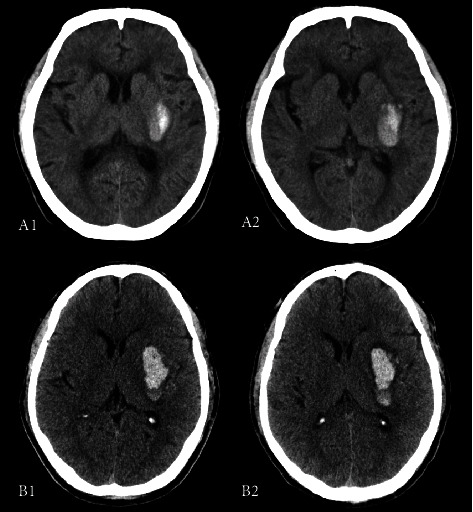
Basal ganglia hemorrhage. Patient A is a 59-year male with left basal ganglia hemorrhage, the baseline CT (A1) scan was performed 40 minutes after symptom onset and 24 hours later, the hemorrhage increased by 3.68 ml (A2), and the mRS was 2 at day 30. Patient B is a 54-year male, the baseline CT (A1) scan was performed 2 hours after symptom onset, and hemorrhage volume was 14.08 ml; the follow-up CT (B2) showed that hemorrhage increased by 3.41 ml (24.22%), and mRS was 4 at day 30.
